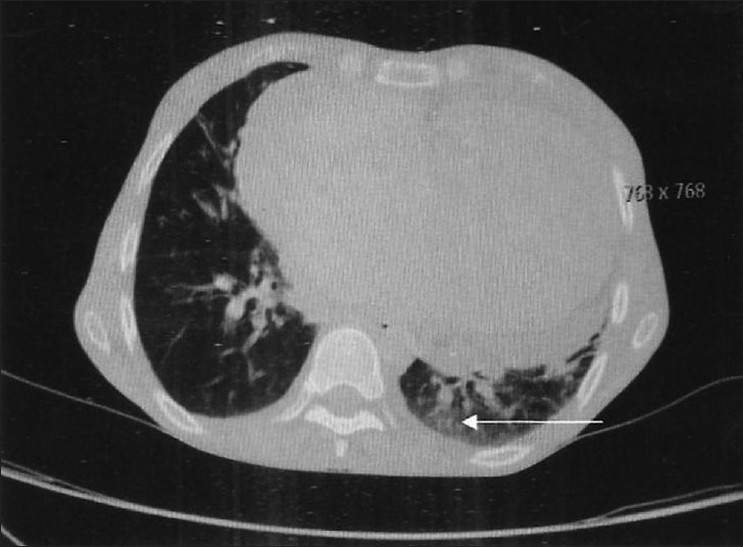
HRCT thorax showing ground glass opacity suggestive of interstitial lung disease
